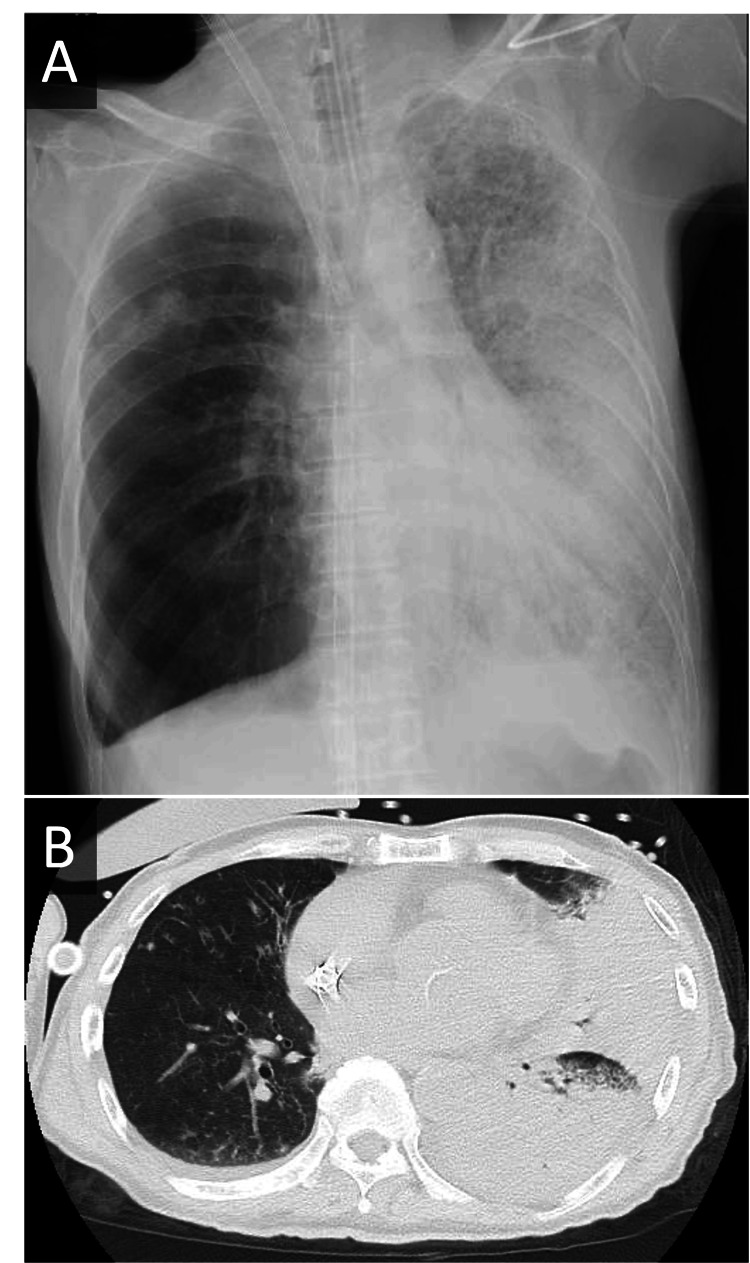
Chest Radiograph and Non-Contrast CT Scan Following Initiation of V-V ECMO (A) Chest radiograph and (B) non-contrast chest CT scan obtained after the initiation of veno-venous extracorporeal membrane oxygenation (V-V ECMO) support, demonstrating extensive infiltrative shadows throughout the left lung.
